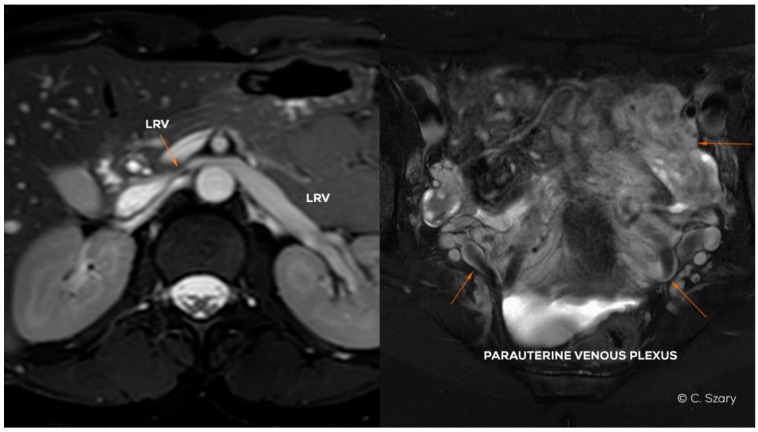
Magnetic resonance (MR) images (BTFE and T2-weighted sequences in axial plane) presenting LRV partial hypoplasia and secondary para-uterine varices caused by LOV insufficiency.
